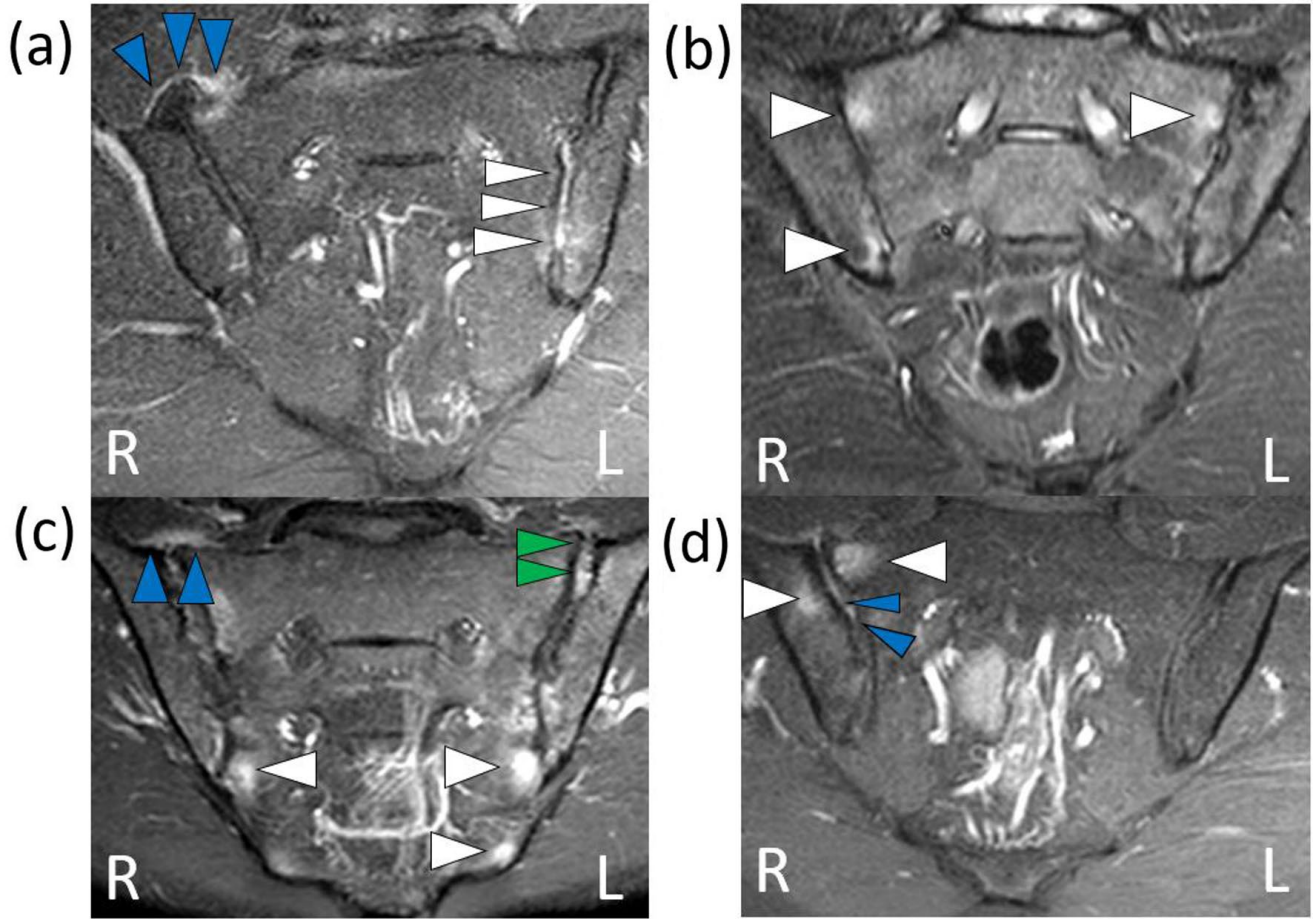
Findings compatible with acute sacroiliitis on MRI of the sacroiliac joints. Source: the authors (2019). Coronal STIR sequence: high signal intensity consistent with synovitis (white arrows) on the left and capsulitis (blue arrows) on the right (a). Coronal STIR sequence: high signal intensity bilaterally consistent with bone marrow edema (white arrows) (b). Coronal T1 post-contrast with fat suppression: high signal intensity bilaterally consistent with bone marrow edema (white arrows) and suggestive of capsulitis (blue arrows) on the right and enthesitis on the left (green arrows) (c). Coronal STIR sequence: high signal intensities on the right compatible with bone marrow edema (white arrows) and enthesitis (blue arrows) (d). R: right side, L: left side.
